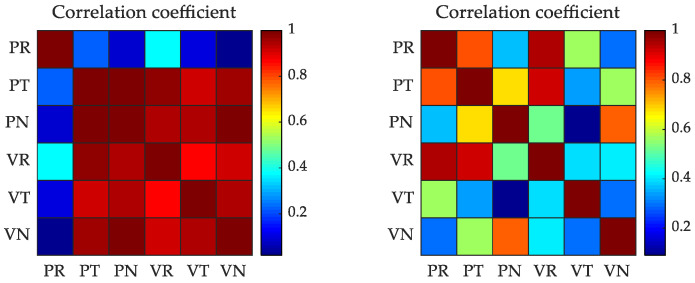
The correlation coefficient of the initial spacecraft states computed with different data (Left: Doppler data, Right: Doppler and image data). “R”, “T”, and “N” denote radial, tangential, and normal directions, respectively. (P: position, V: velocity).
